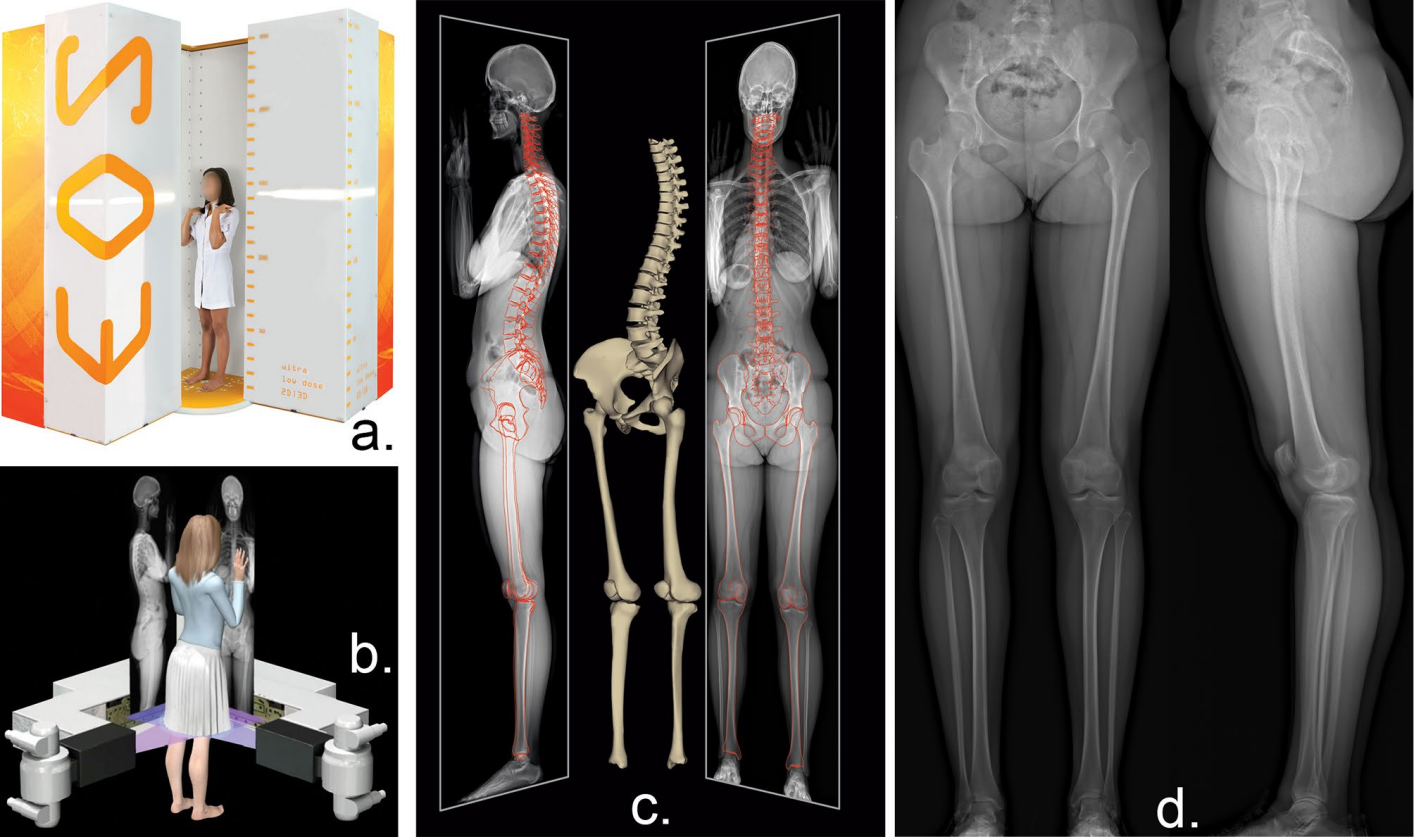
The EOS 2D/3D Imaging System. (a) The EOS 2D/3D Imaging System and the typical positioning during examination (source: www.eos-imaging.com). (b) The basic operation of the imaging system: upright biplanar slot-scanning X-ray imaging (source: www.eos-imaging.com). (c) 3D reconstruction opportunities of the lower limb and spine (source: www.eos-imaging.com). (d) Example of a typical EOS 2D/3D lower limb image-pair used in this study.
